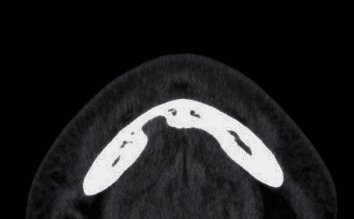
Computed tomography (CT) image, horizontal cross section, soft tissue mode. The inside of the cavity in the lingual side of the mandible has similar soft tissue density (CT number, 40–70 Hounsfield units (HU)) to the sublingual glands (CT number, 40–70 HU), with findings that suggest continuity with the sublingual glands.
